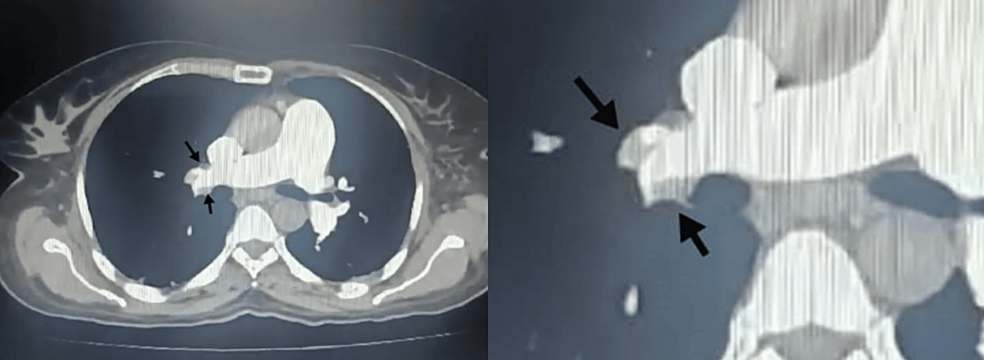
CTPA of the patient The black arrows show the right upper lobe pulmonary artery thrombus CTPA: computed tomography pulmonary angiogram
